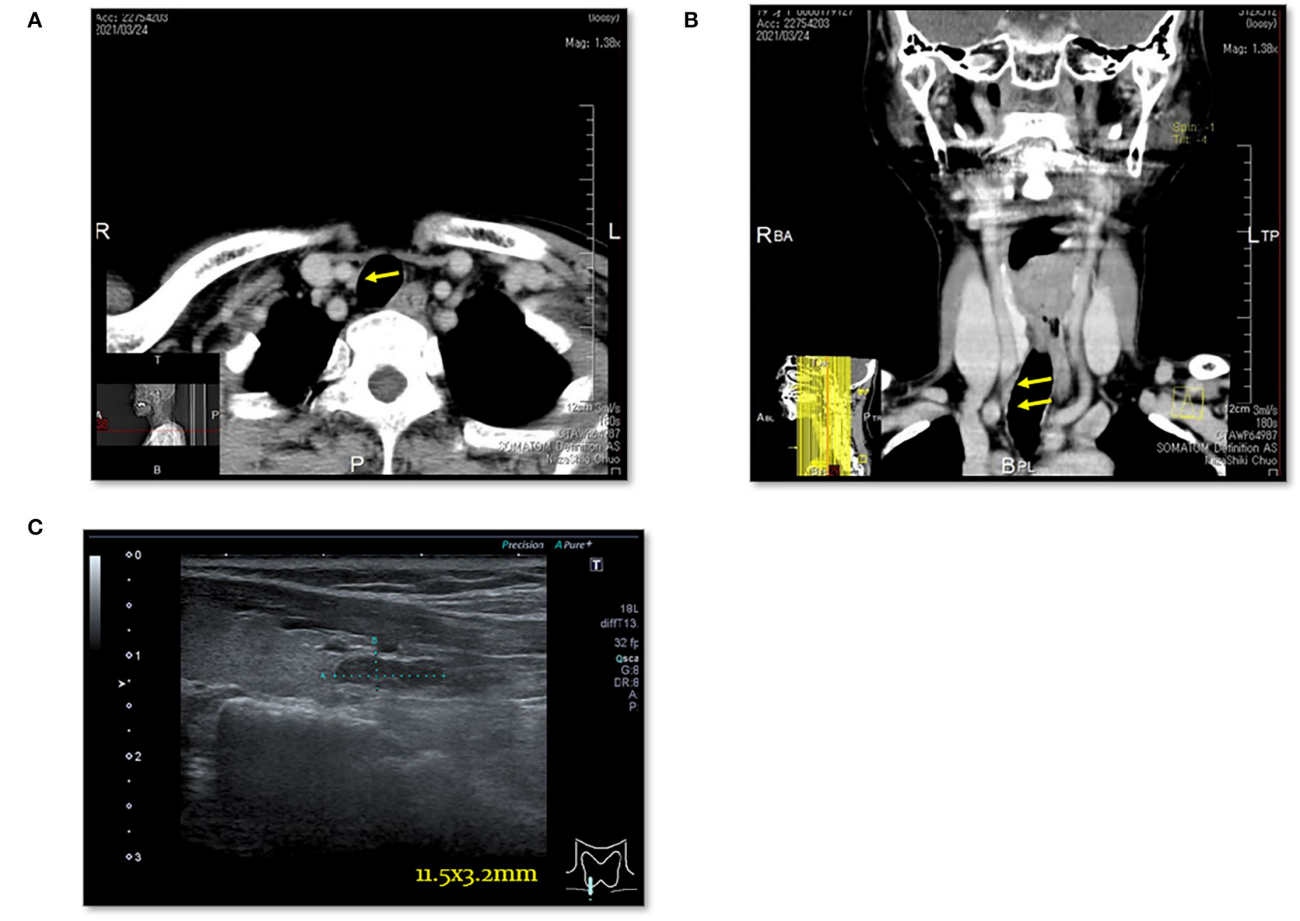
Case 8. (A) Thoracic enhanced CT scan showing a mass (EPA: yellow arrow) near the right trachea. (B) Thoracic enhanced CT (MPR) scan showing a mass (EPA: yellow arrow) near the right trachea. (C) Cervical neck ultrasound showing a mass [11.5 × 3.2 mm (EPA)] near the cervical esophagus.
